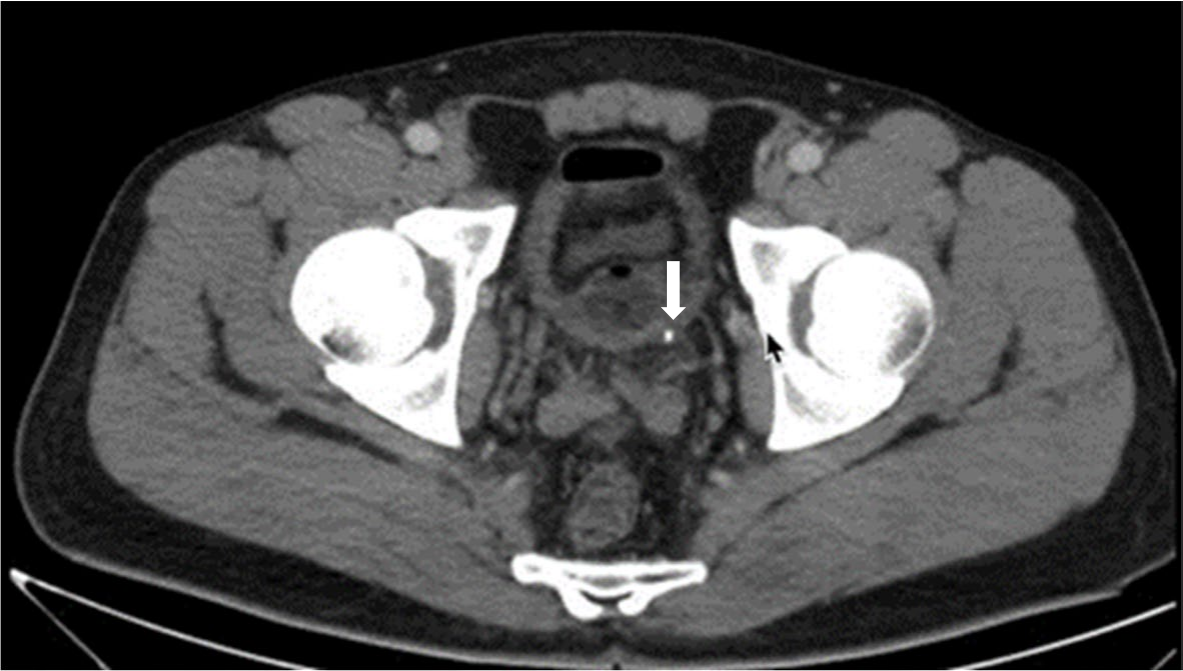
Abdominopelvic CT scan with contrast showing a small stone in the ureterovesical junction
